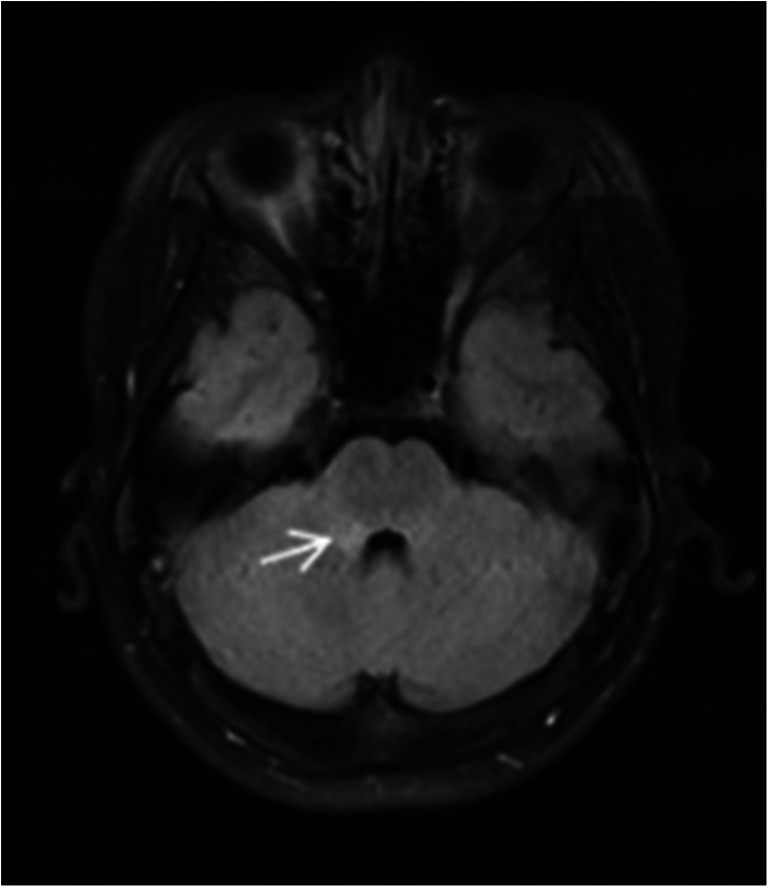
case 1: Brain MRI shouwed lesion in right brachium pontis on Flair on admission
